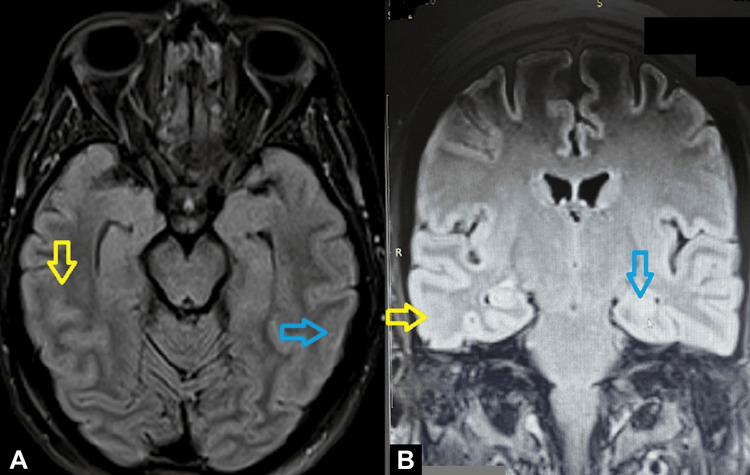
(A, B) Patient's magnetic resonance imaging (MRI). Yellow arrows demonstrate mild edema/unclarities of the right temporal lobe. Blue arrows demonstrate mild edema/unclarities of the left temporal lobe. MRI descriptions may be vague when abnormalities are subtle, evolving, affected by technical limitations, or when the underlying disease, such as encephalitis, frequently produces non-specific or even normal imaging findings, especially early in its course.
